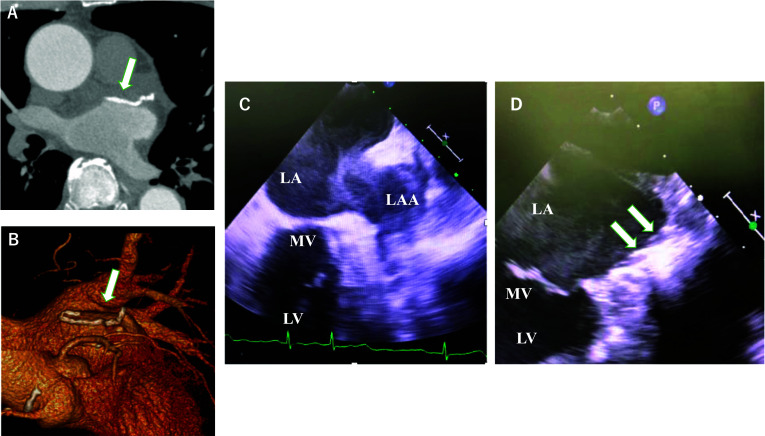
Post-operative contrast-enhanced computed tomographic images. A, B Case 1. The LAA was resected from the base (arrows). C, D Case 2. Transesophageal echocardiograph images obtained before (C) and after (D) left atrial appendage closure. *LA* left atrium, *LV* left ventricle, *MV* mitral valve
